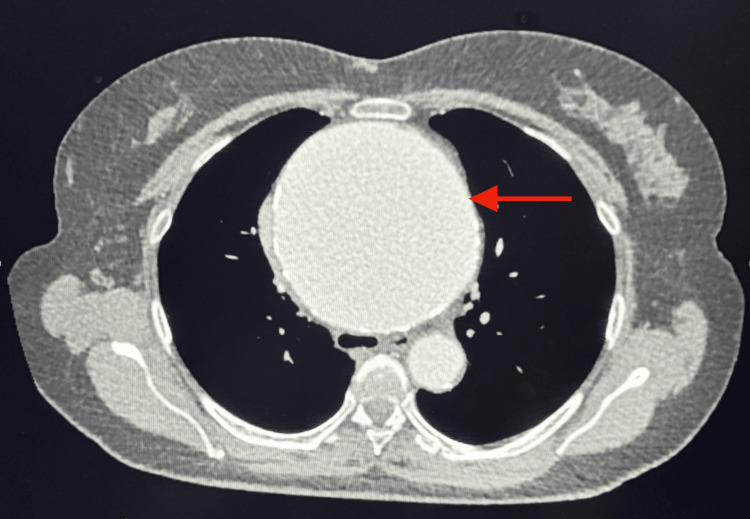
Transverse section of a thoracic CT scan demonstrating a giant ascending aortic aneurysm (red arrow).
